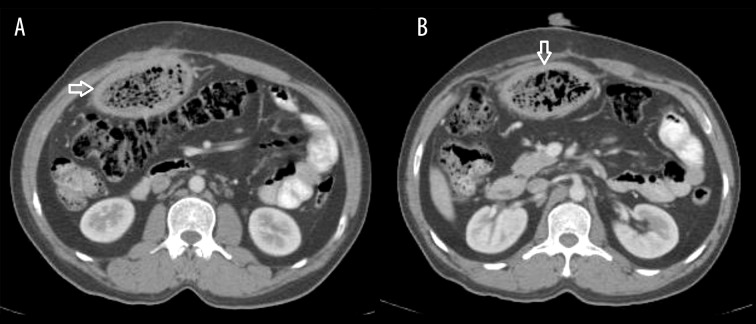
(A, B) Enhanced CT scan of the abdomen reveals a gas-containing, spongiform mass adherent to the anterior abdominal wall.
